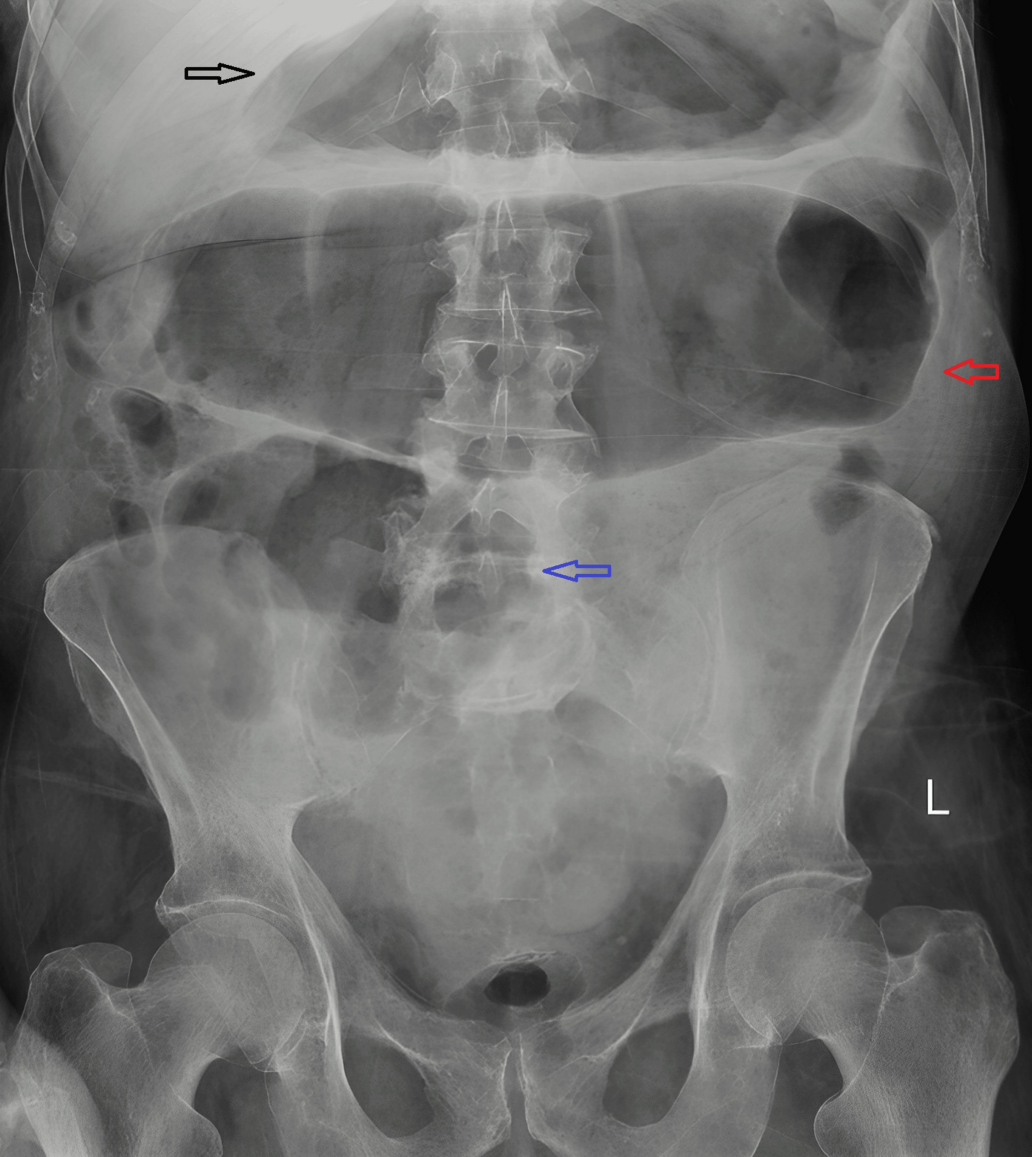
Abdominal X-ray on day three. Dilated transverse colon measuring up to 9.7 cm in keeping with megacolon (red arrow), with gassy distension of the stomach (black arrow) and the caecum (blue arrow).
